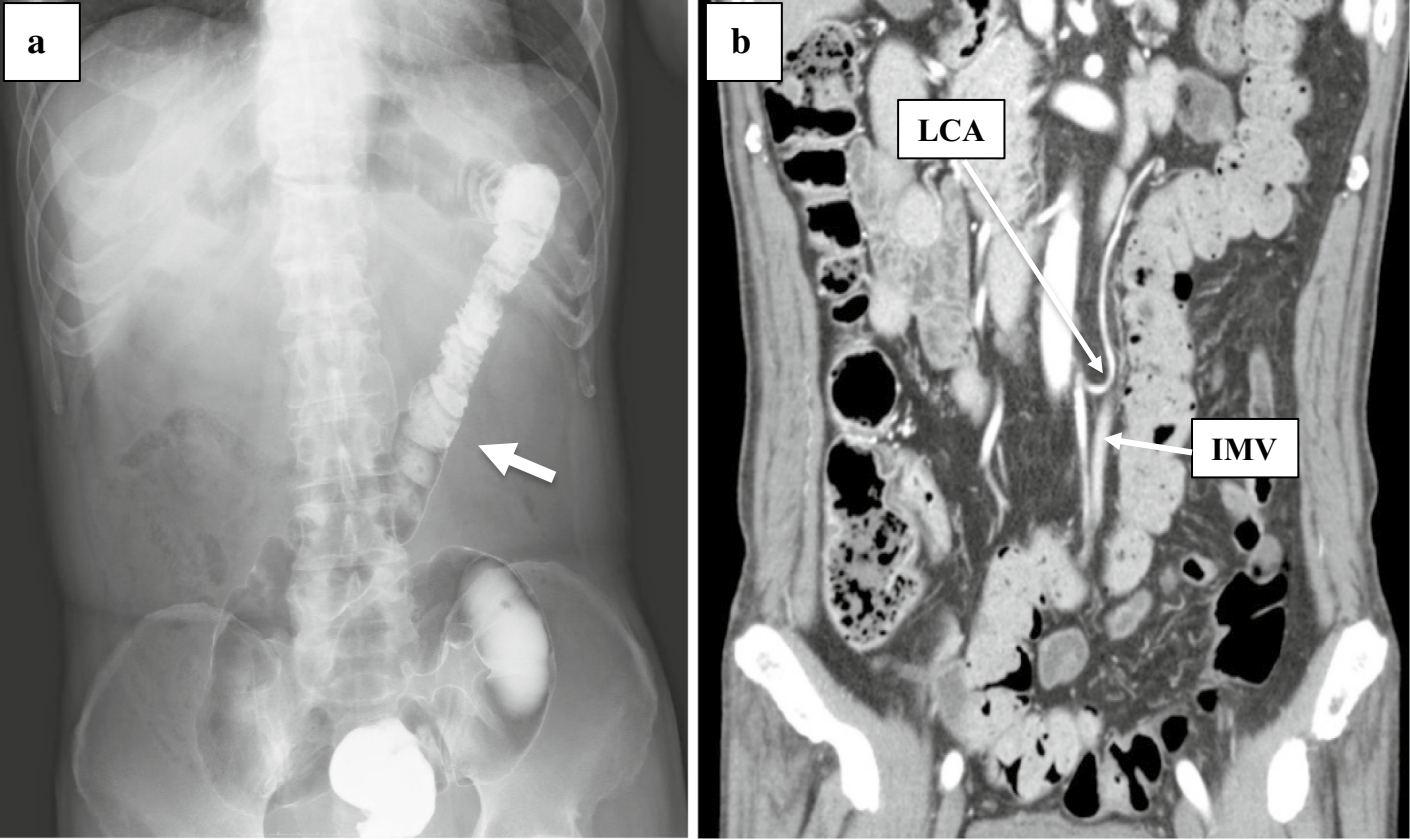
Case 3. a BE revealed moderate displacement of the descending colon to the midline (white arrow). b An enhanced CT showed shortening of the descending mesocolon. LCA, IMV, and marginal vessels ran very close to each other
